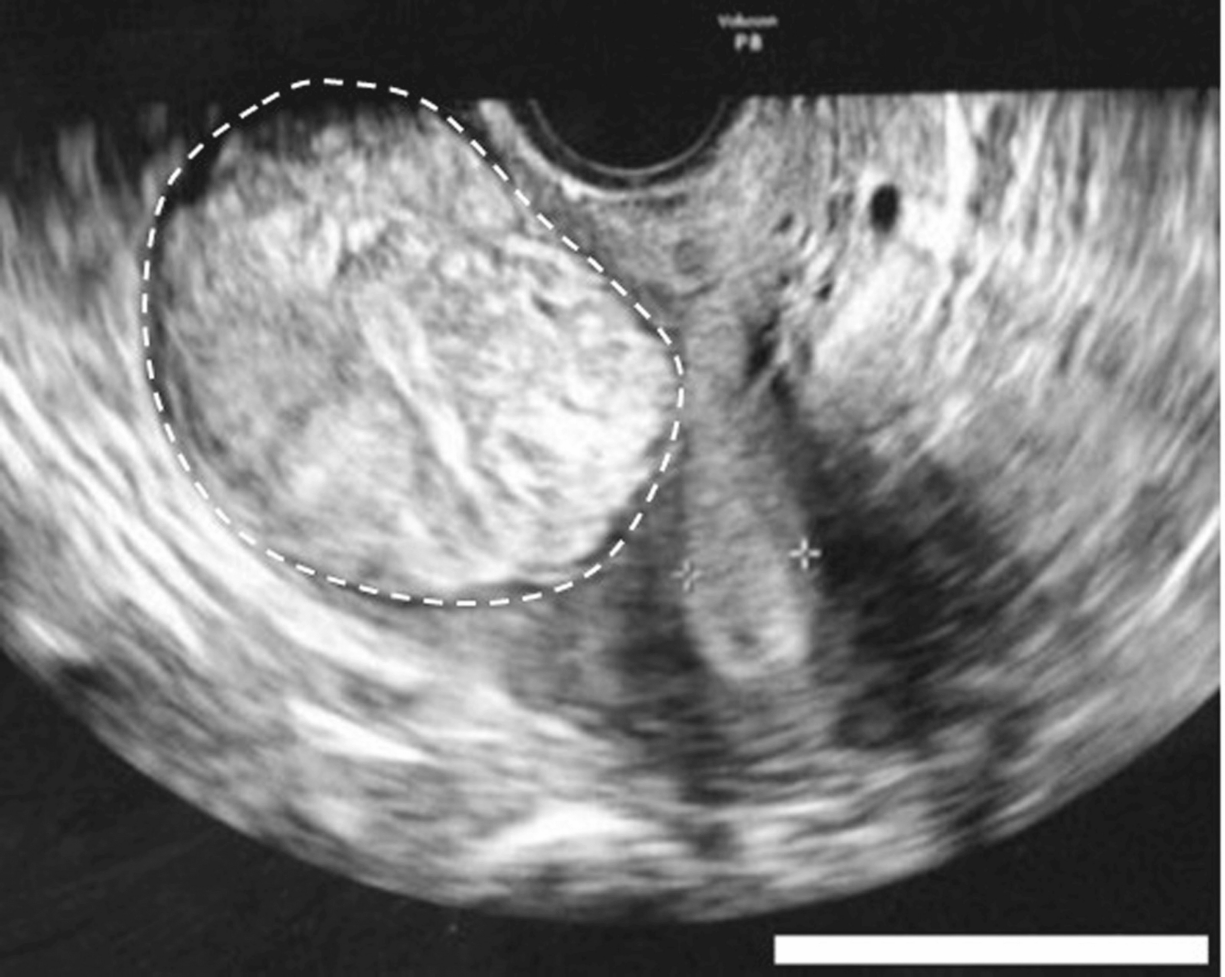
Transvaginal ultrasonography at the initial visit shows a 6 cm uterine myoma in the pouch of Douglas Scale bar is 5 cm.
